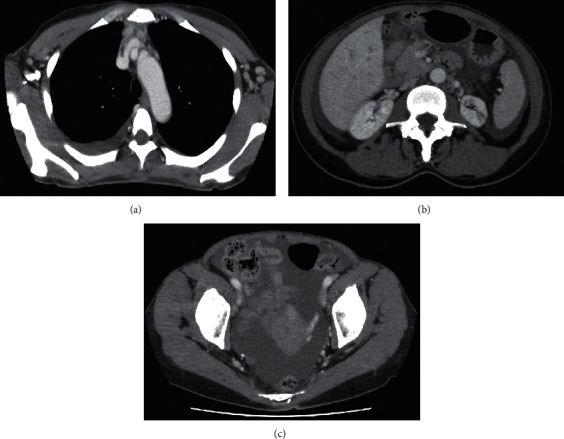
Computed tomography revealing enlargement of the axillary lymph nodes and right pleural effusion (a) and ascites around the liver and spleen (b) and in the pelvic cavity (c).
